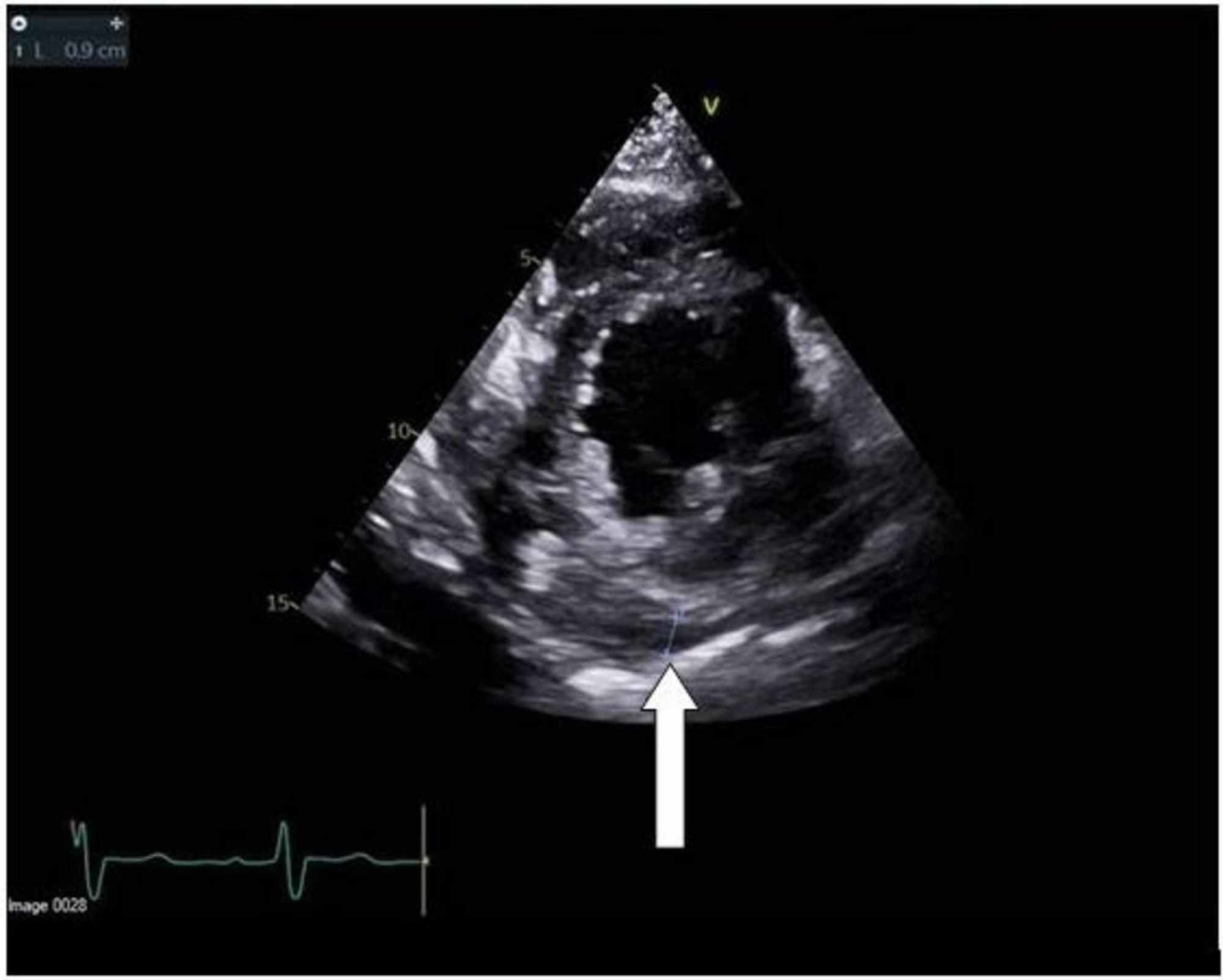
PSAX view of echocardiogram demonstrating thrombosed pseudoaneurysm PSAX: parasternal short axis
